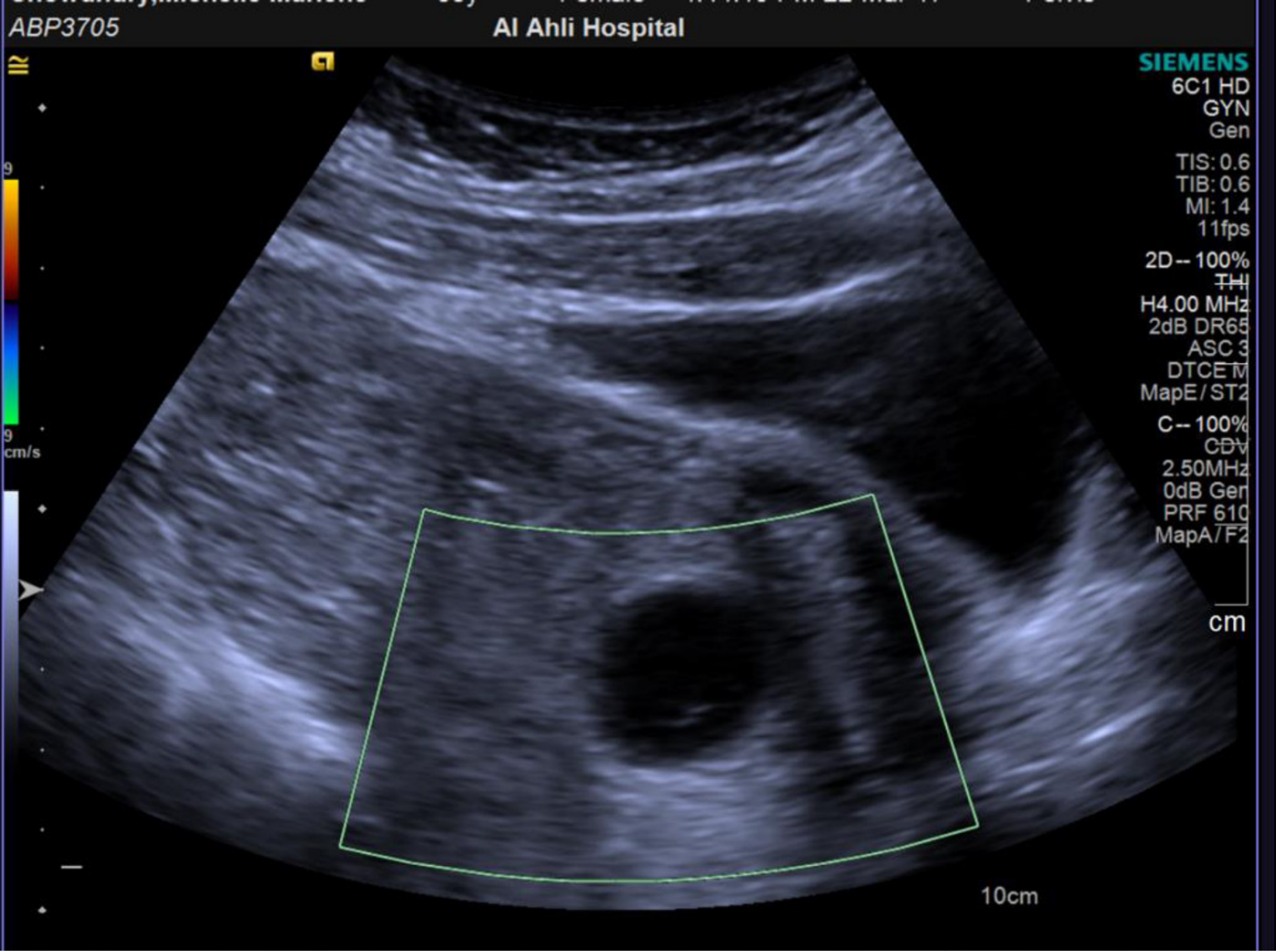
Absent vascularity on color Doppler.
